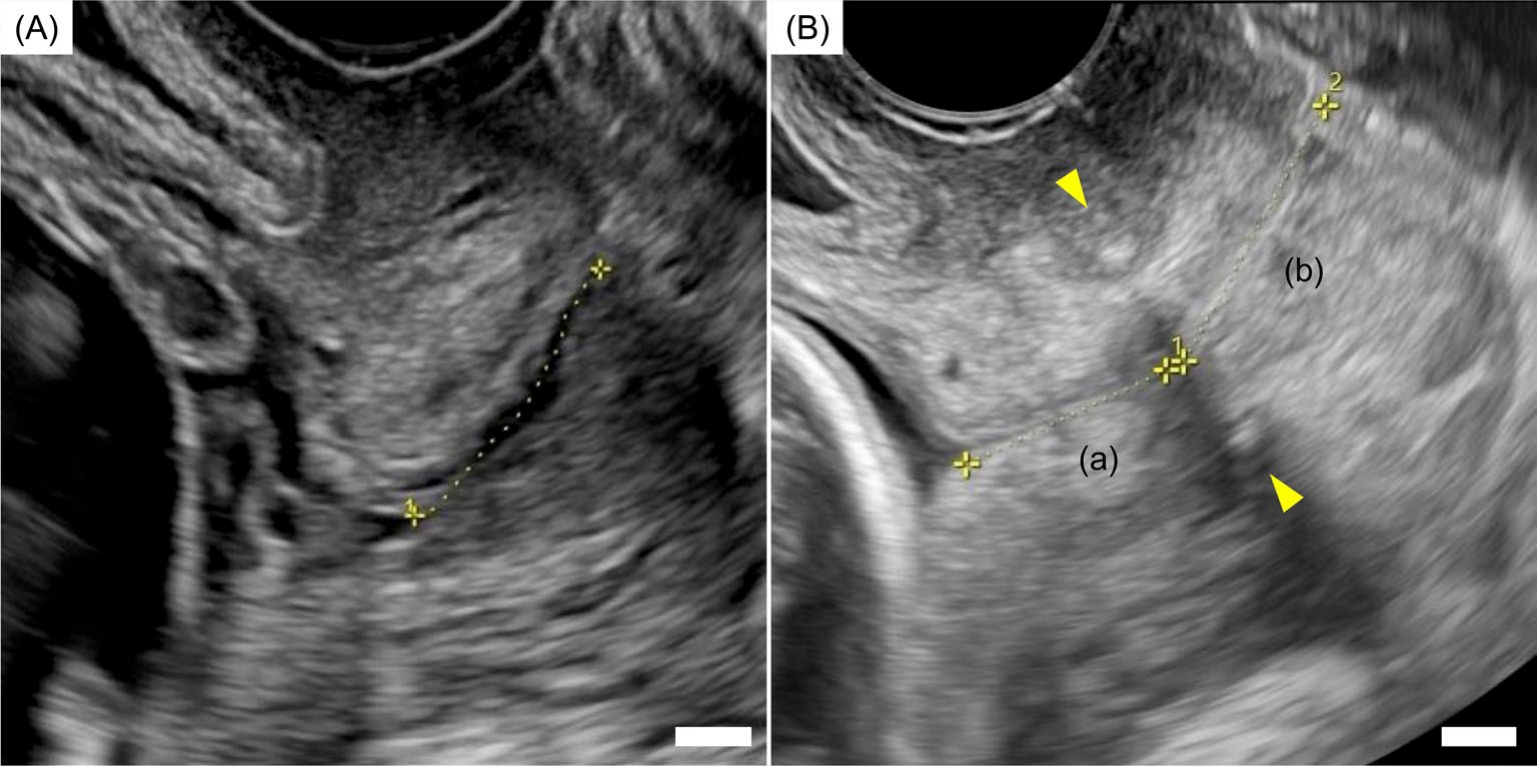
Transvaginal ultrasonography findings during pregnancy. (A) Transvaginal ultrasonography before cervical cerclage. The yellow line represents the total cervical length, measured at 20.5 mm. (B) Transvaginal ultrasonography after cervical cerclage. The yellow line labeled “(a)” indicates the distance from the internal os to the Shirodkar cerclage tape (14.7 mm), while the line labeled “(b)” indicates the distance from the external os to the cerclage tape (19.5 mm). The yellow arrowheads indicate the location of the Shirodkar cerclage tape. The white bars indicate 5 mm.
